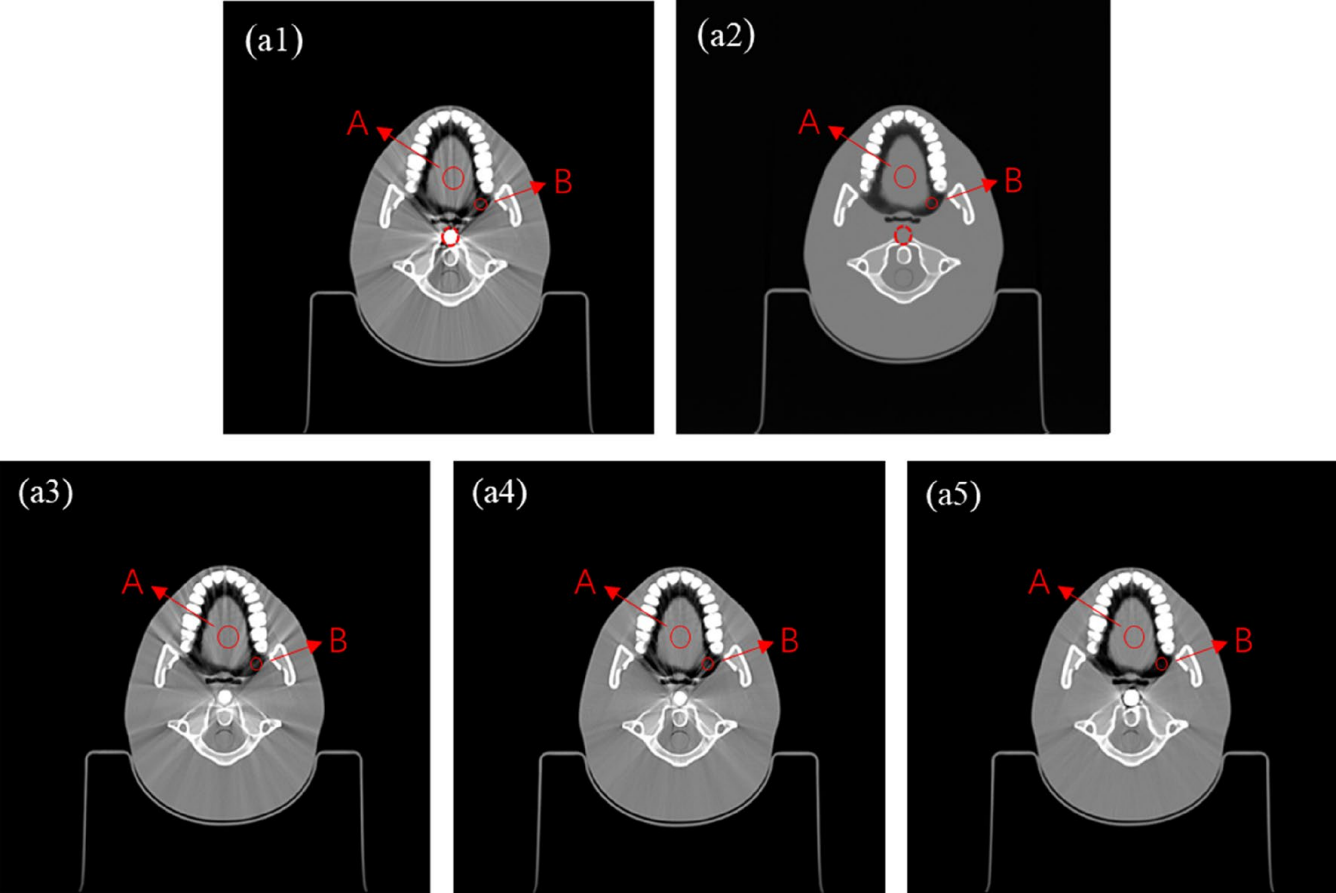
The origin and results of images corrected by different methods: (a1) Original kV-CT Image; (a2) Reference image without metal; (a3) LIMAR method; (a4) NMAR method; (a5) Proposed method.
